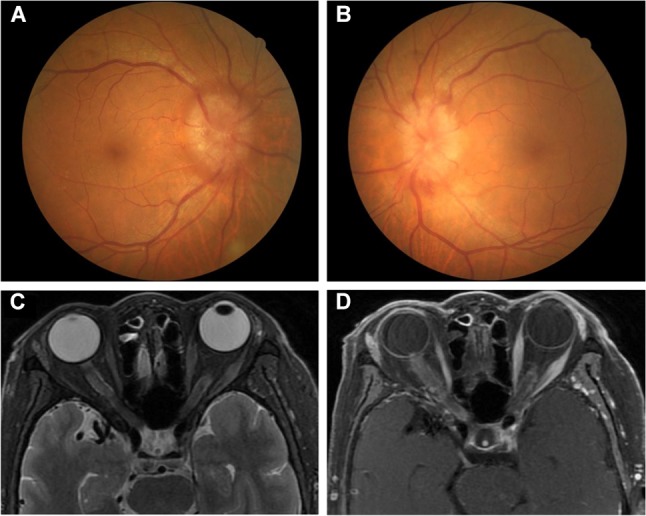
(A,B) Fundus examination in Case 2. Bilateral optic disc oedema. (C,D) Orbital MRI in Case 2. (C) Bilateral optic nerve enlargements of orbital and inner canal segments in T2-weight imaging. (D) Bilateral leptomeningeal enhancement in optic nerves when gadolinium was administered (“tram-tracking” sign).
